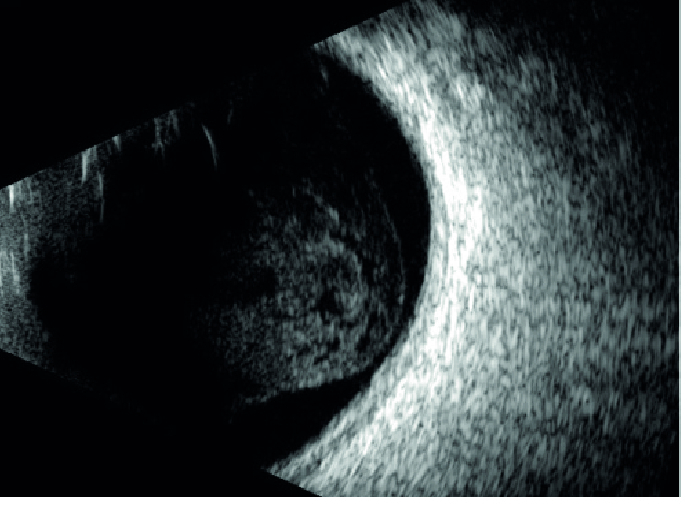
B-scan ultrasound 16 months after proton beam radiation shows complete posterior vitreous detachment and moderate point-like echodensities in the posterior vitreous cavity.
